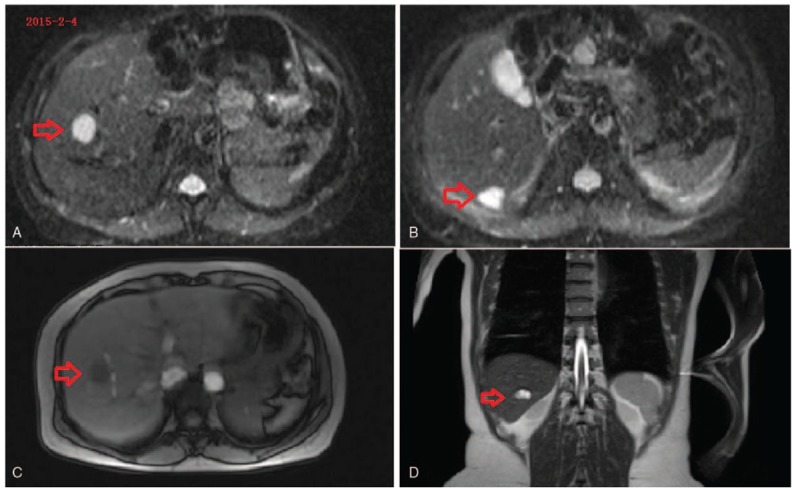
Contrast-enhanced magnetic resonance imaging showing multiple masses in the liver (red arrow). (A) Hemangioma in right anterior lobe; (B) hemangioma in right posterior lobe; (C) hemangioma in right anterior lobe; (D) hemangioma in right posterior lobe.
